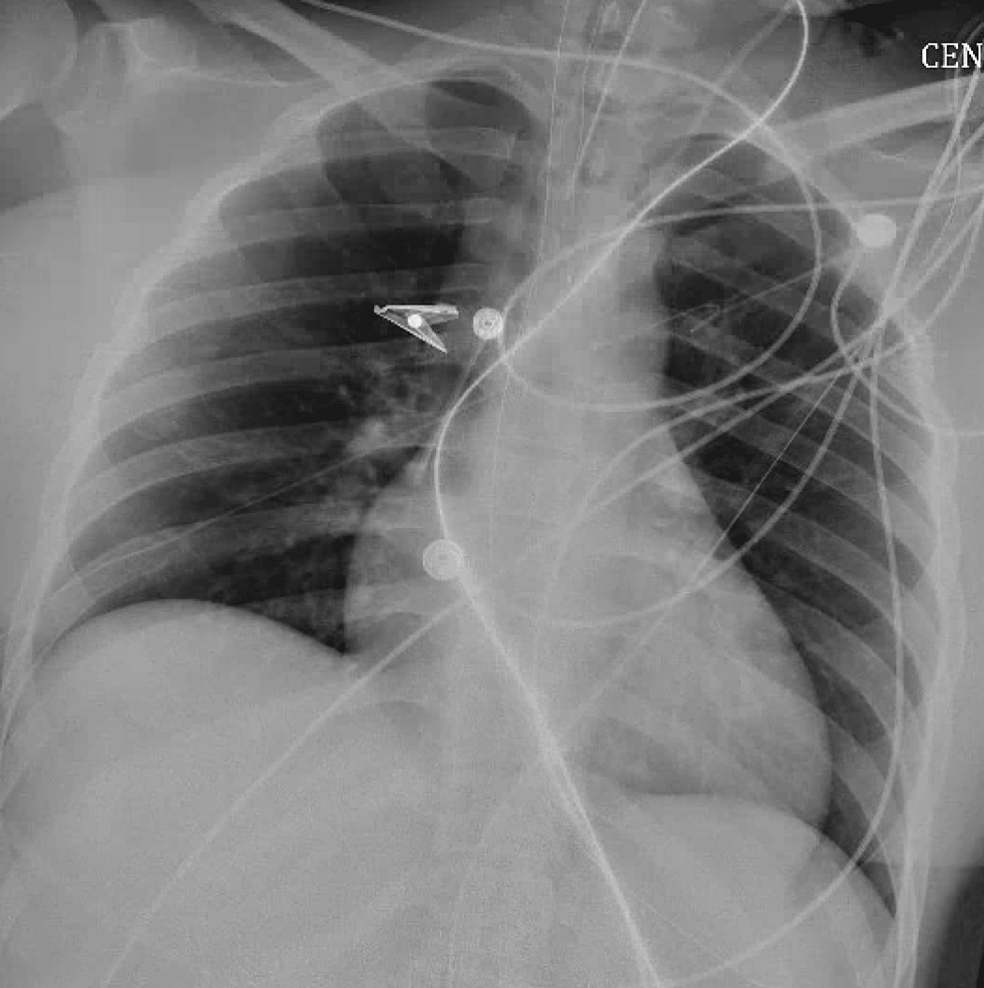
A chest X-ray taken shortly after the patient's arrival at the hospital, following endotracheal intubation and the insertion of a central line The chest X-ray showed no signs of acute abnormalities.
